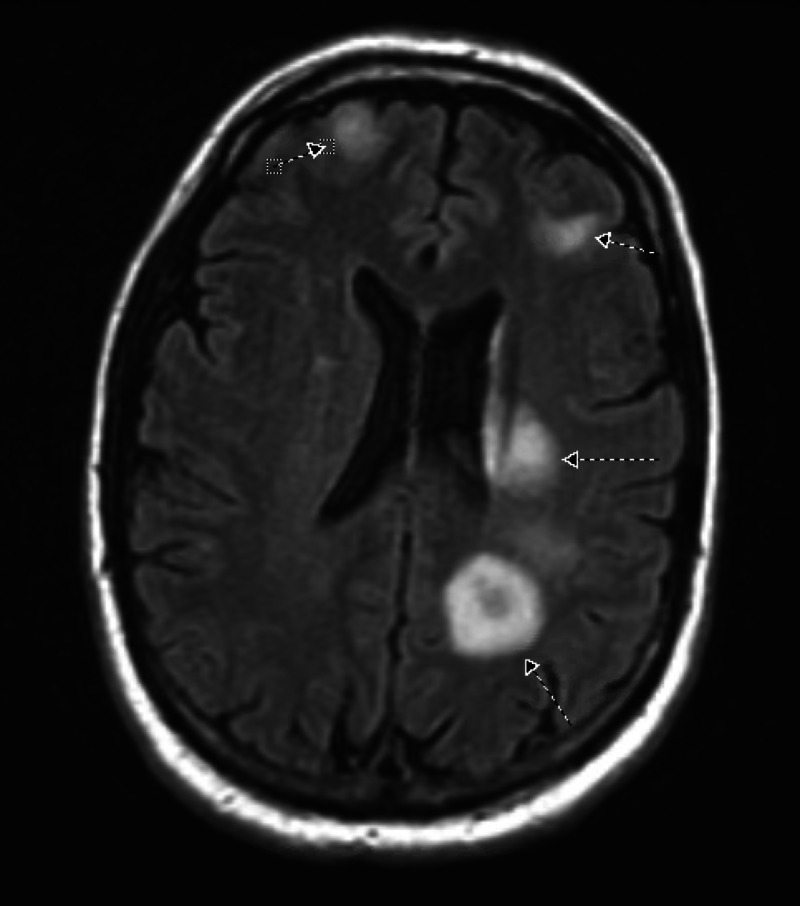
MRI of the brain Arrows indicate multiple, bilateral ring-enhancing lesions with more lesions on the left cerebrum than the right cerebrum MRI: magnetic resonance imaging
